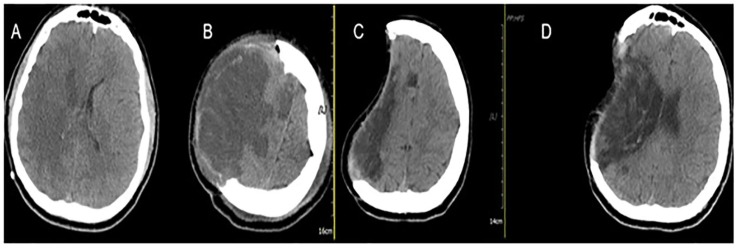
Computed tomography (CT) scans of a 69-year-old male patient with right-sided MMCAI (A). Postoperative CT shows the wide craniectomy region (B). After two months, CT showed favorable results without midline shift (C,D).
